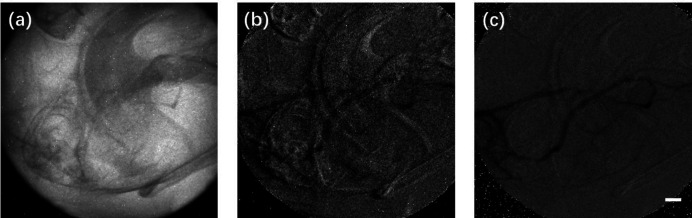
Experimental results obtained using an X-ray tube. (*a*) The primary projection of the cerebral microvessels. (*b*) Image obtained using the conventional temporal subtraction method. (*c*) Image obtained using the PTA method. Scale bar: 1 mm.
